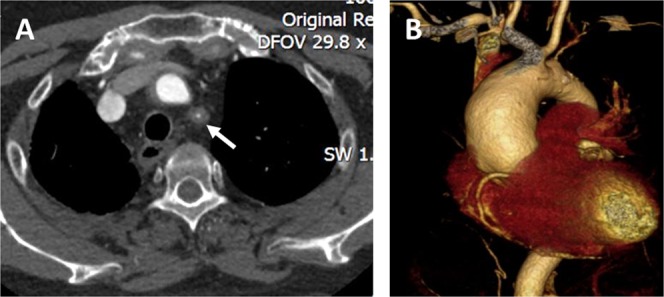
Computed tomography angiography of the aorta of a Takayasu arteritis patient. A 50-year-old female patient was diagnosed with Takayasu arteritis for 20 years. (A) Obvious thickening of the vascular wall leading to severe stenosis in the left subclavian artery (white arrow). (B) Aneurysm formation in the ascending aorta.
